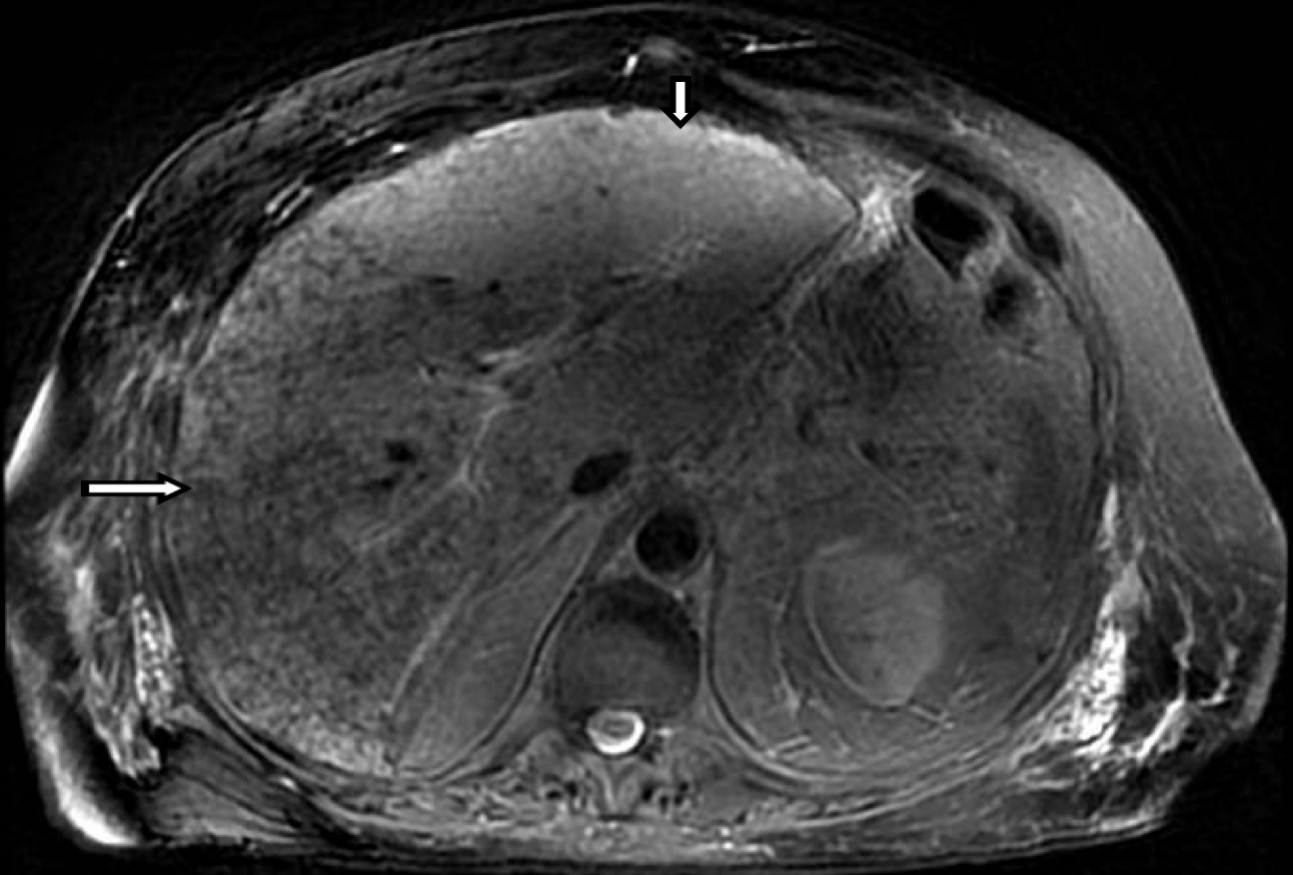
Magnetic Resonance Imaging (MRI) of the Abdomen without contrast T2 weighted axial image at the same level of the liver demonstrates abnormal heterogeneous increased T2 signal predominately involving the left hepatic lobe (small arrow). There are small areas of normal liver seen (long arrow), suggesting an infiltrative process.
